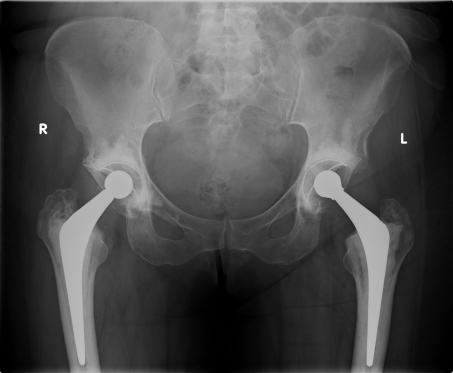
X-ray pelvis following total hip replacement.
